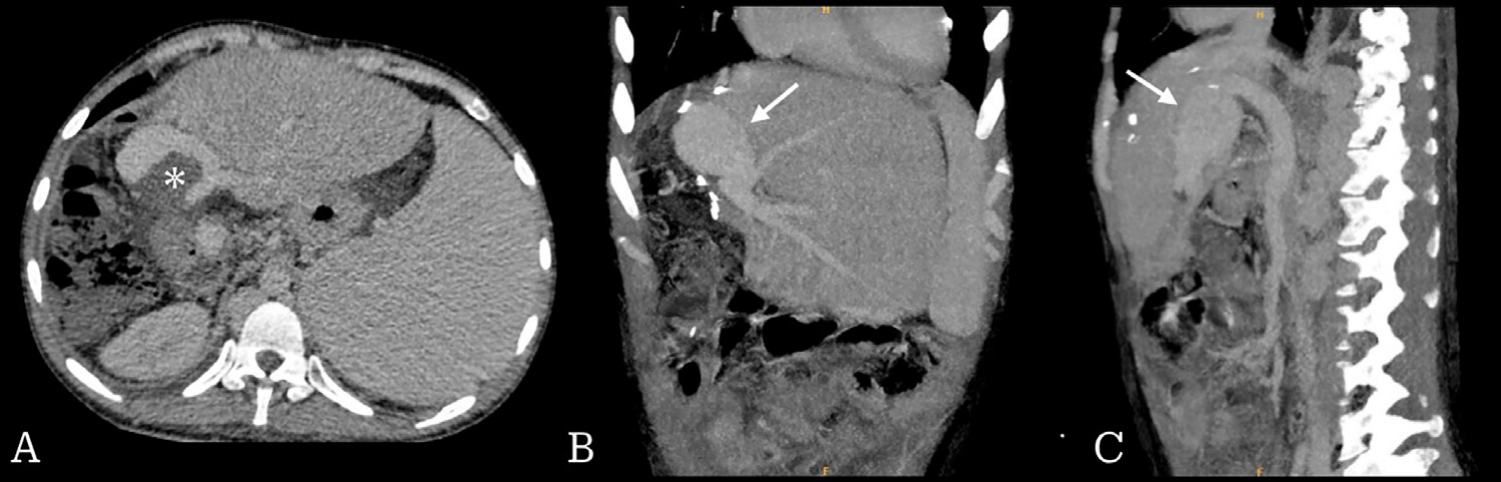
Contrast-enhanced abdominal CT: (A) Axial view showed thrombus (asterisk) in the main portal vein. (B) Coronal and (C) sagittal view showed varix in the main portal vein (arrow).
